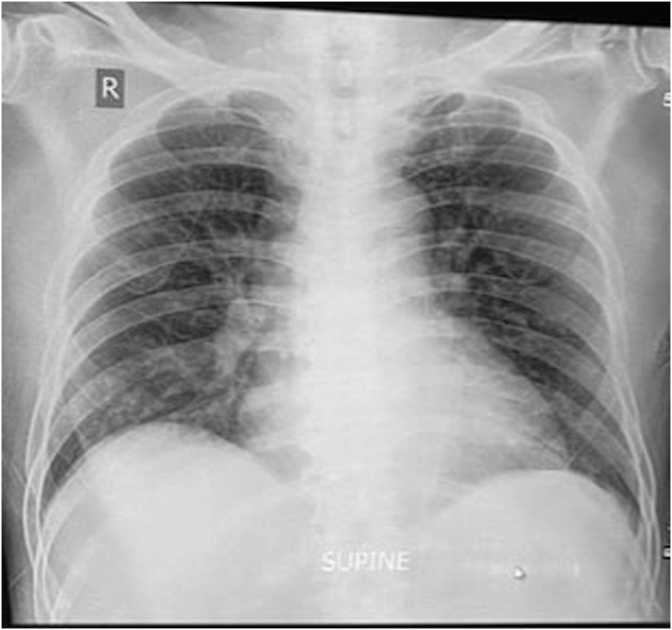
Plain chest X-ray was within normal limits.
